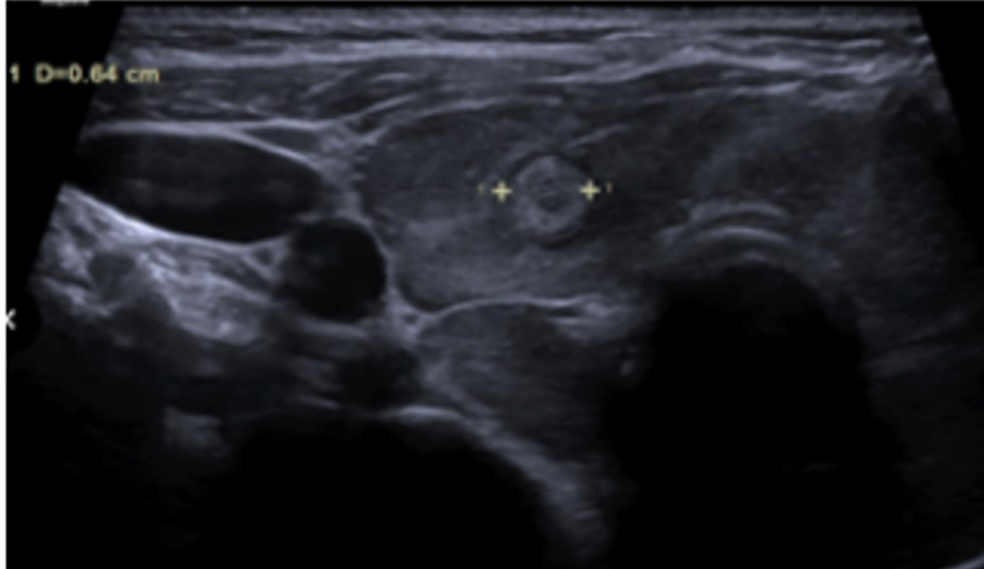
Ultrasound of thyroid. Heterogeneous thyroid echogenicity with increased parenchymal vascularity.
